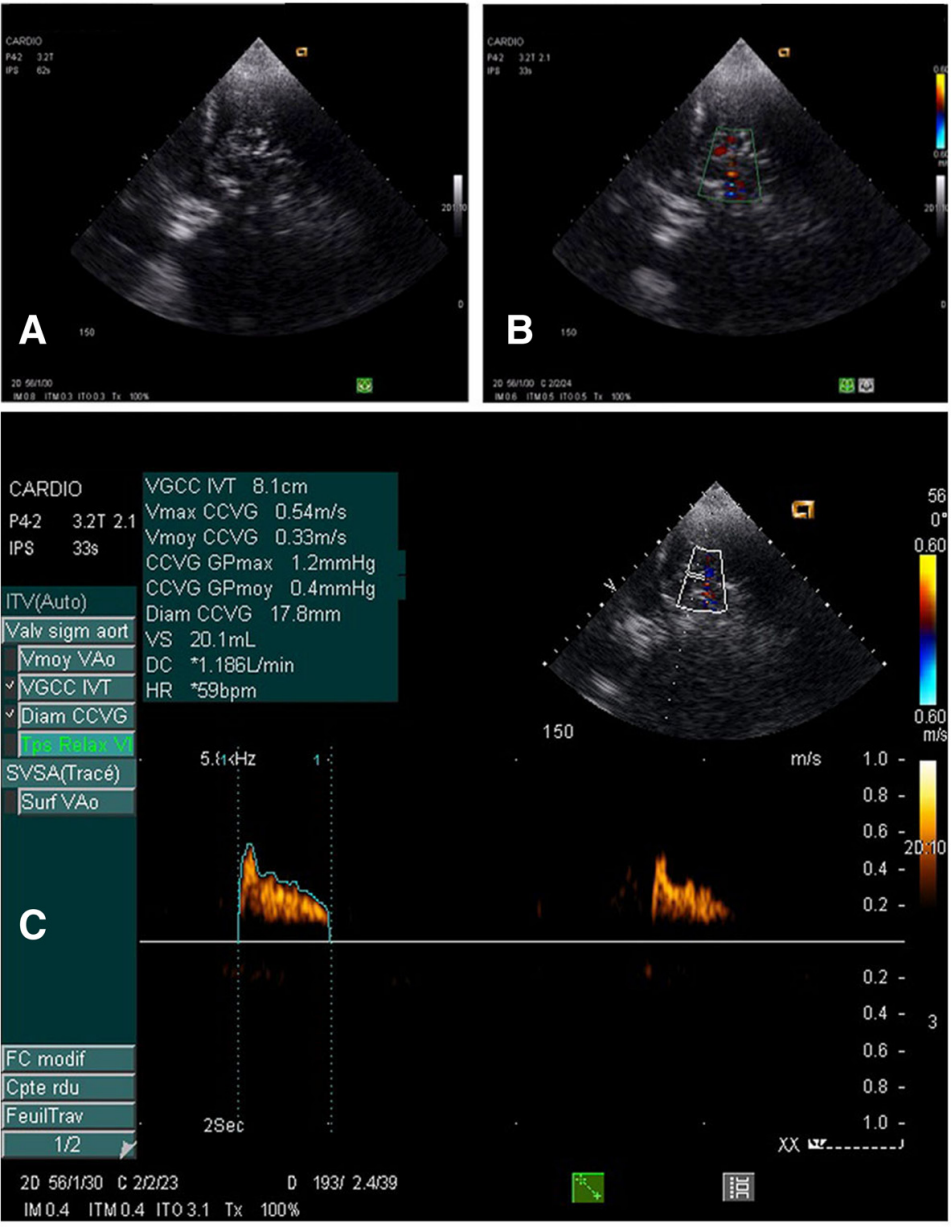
Pulmonary blood flow. (A) Lung consolidation with persisting regional pulmonary blood flow (B) detected using transthoracic Doppler ultrasound in a patient included in the study. Within the consolidation, a pulmonary artery characterized by its biphasic signal is identified (C).
